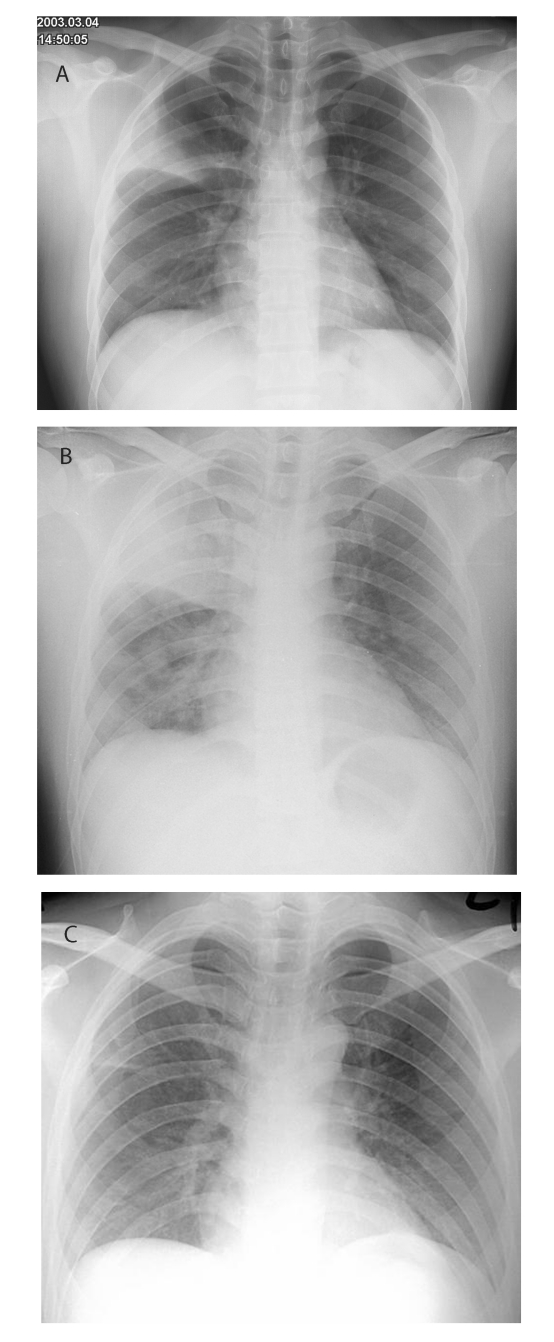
Chest radiographs performed A, at admission, B, on day 4, and C, on day 16 of hospitalization for index SARS case-patient, Prince of Wales Hospital.
